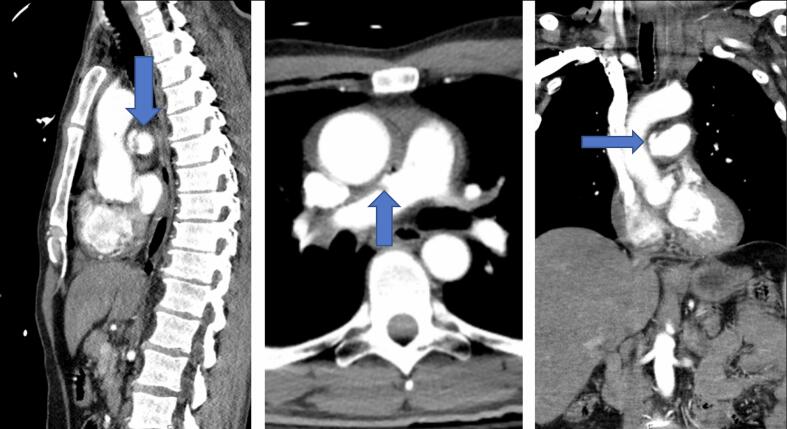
(A) Sagittal, (B) axial, and (C) coronal CT images demonstrating pseudoaneurysm in the proximal right pulmonary artery.
